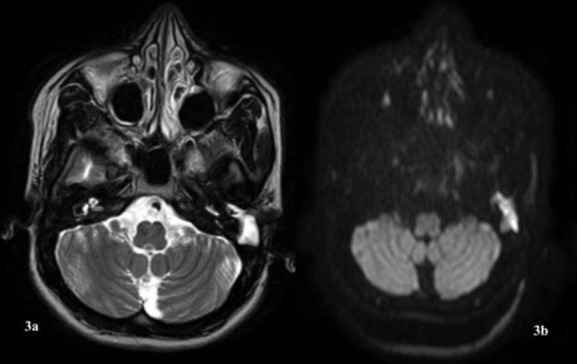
T2W Gadolinium MRI image showing a hyperintense density in the left external auditory canal and mastoid. 3b: DWI MRI image showing a hyperintense density in the left external auditory canal.
